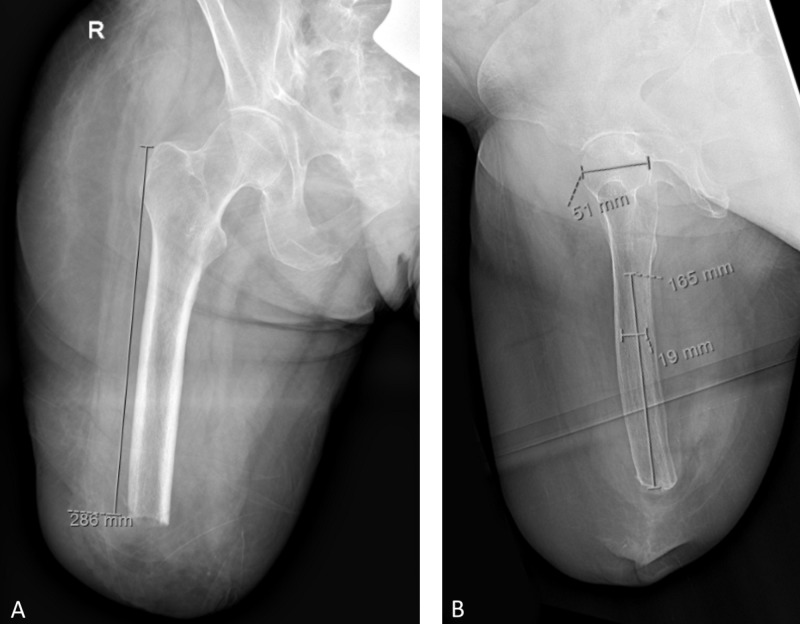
Preoperative radiographs of the right femur. (A) On the anterior-posterior view, the distance from the greater trochanter to the distal femur measured 286 mm. (B) On the lateral view, the distance from the lesser trochanter to the distal femur measured 165 mm and the canal diameter was 19 mm.
